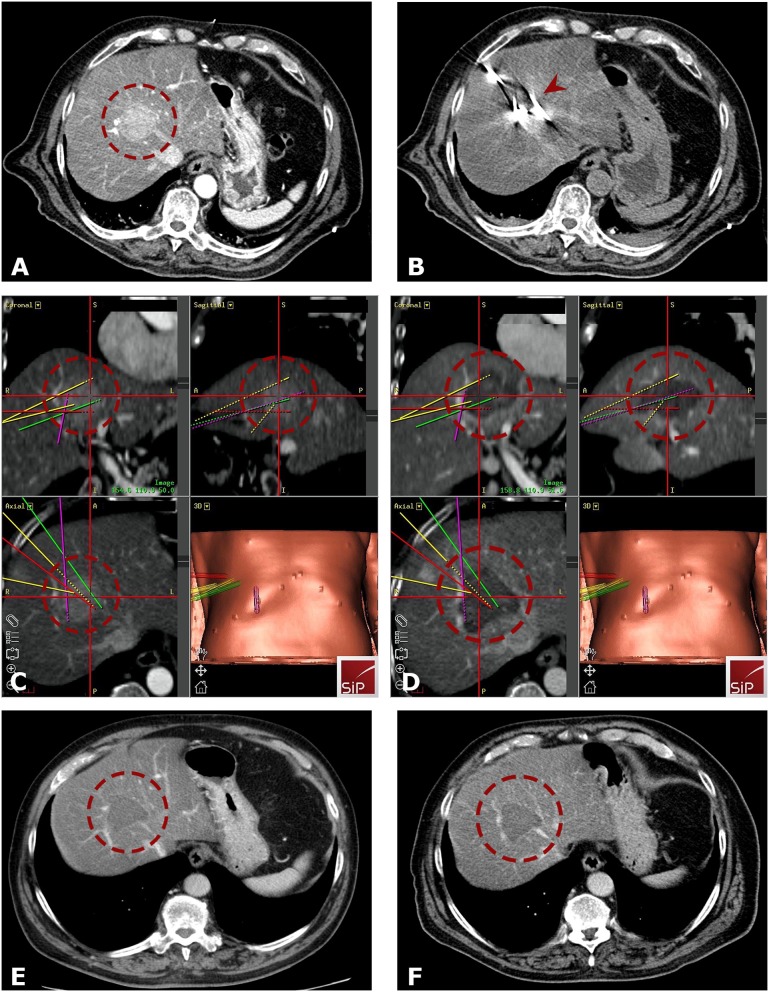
Case of a 81-years old male with a HCC in segment VIII. (A) Arterial phase planning CT with a 4.5 cm HCC close to portal vein and infiltration/obstruction of the middle hepatic vein (*red dashed circle*). (B) Native control CT showing in total 5 inserted coaxial needles (*red arrowhead*). (C,D) Fused images from the navigation system with 3D views from arterial phase planning CT (A) and final control CT (D) with complete necrosis including a sufficient ablation margin (*red dashed circle*). The colored lines correspond to the predefined paths. (E,F) Follow- up CT scans after 18 (E) and 24 months (F) with no evidence of local tumor recurrence (*red dashed circle* is marking the coagulation zone).
